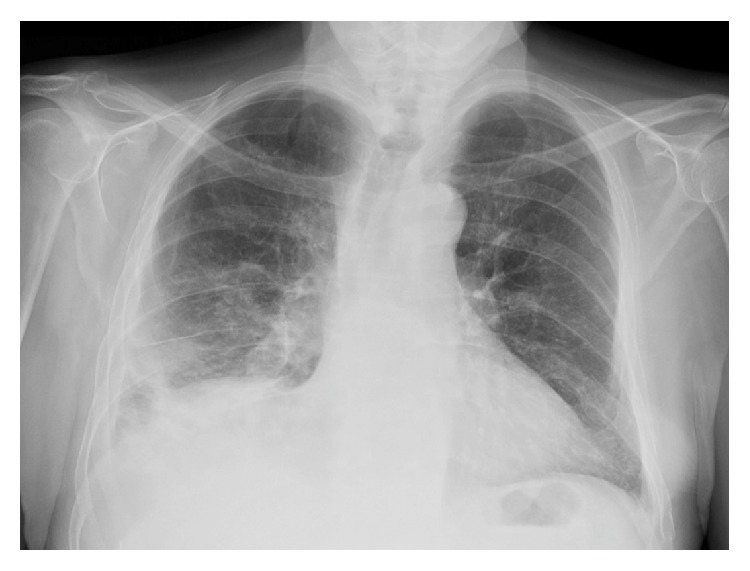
Control chest X-ray. Loss of volumen of the right lung and improvement of the right alveolar basal infiltrate.
